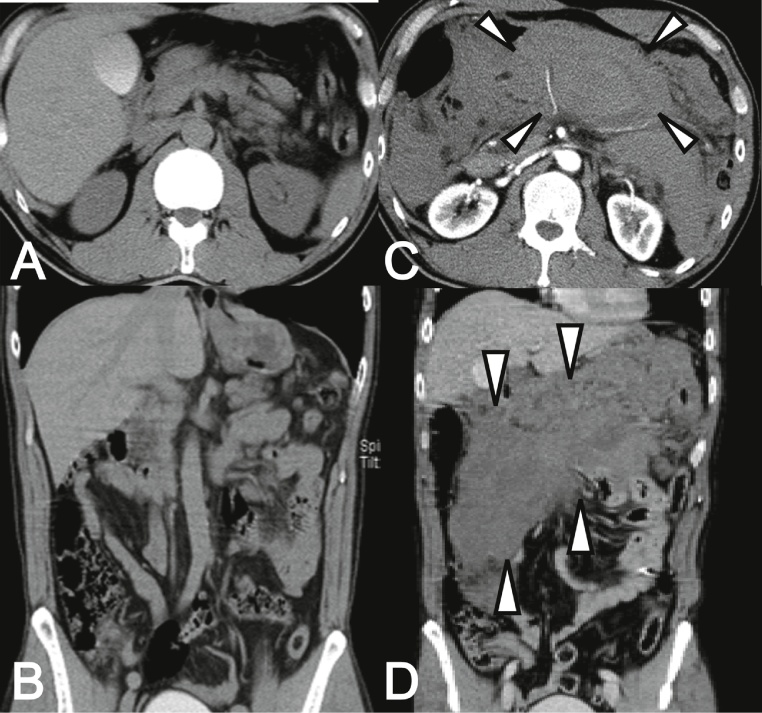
Plain computed tomography (CT) examination on day 2 was unremarkable (A, B). Contrast-enhanced CT examination with rapid fluid infusion, performed immediately after resuscitation from hypovolemic shock, revealed a mass with both high- and low-density areas with a 13 cm maximum diameter bordering the transverse colon (arrowhead, C, D).
